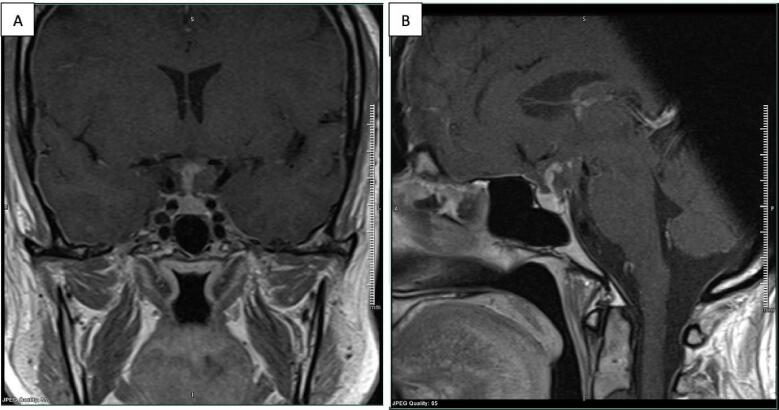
Postoperative MRI findings. Adjacent expected post-operative changes are seen. A small, L-shaped residual enhanced part of the mass is still seen. B It represents the superior and right anterolateral aspect of the mass.
